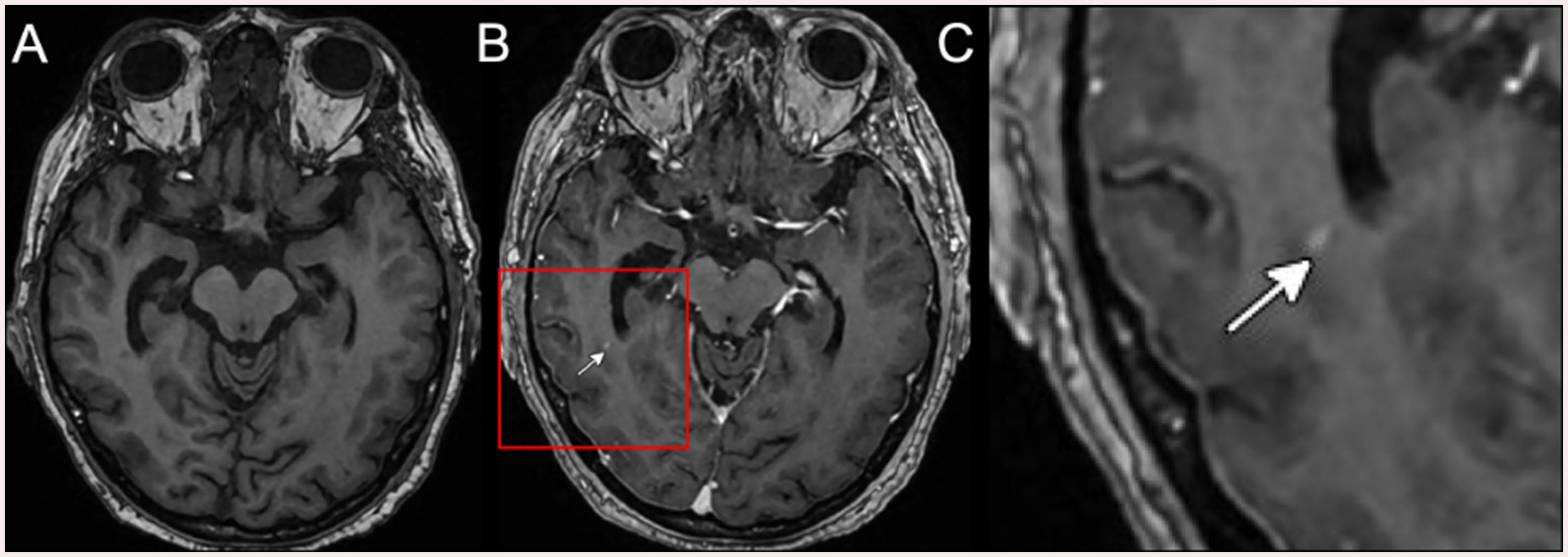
MRI brain on hospital day 3, demonstrating a single punctate focus of contrast enhancement in the right medial temporal lobe. (A) T1 pre-contrast sequence, (B) T1 post-contrast sequence, and (C) magnified image of the T1 post-contrast image region of interest shown in panel (B).
